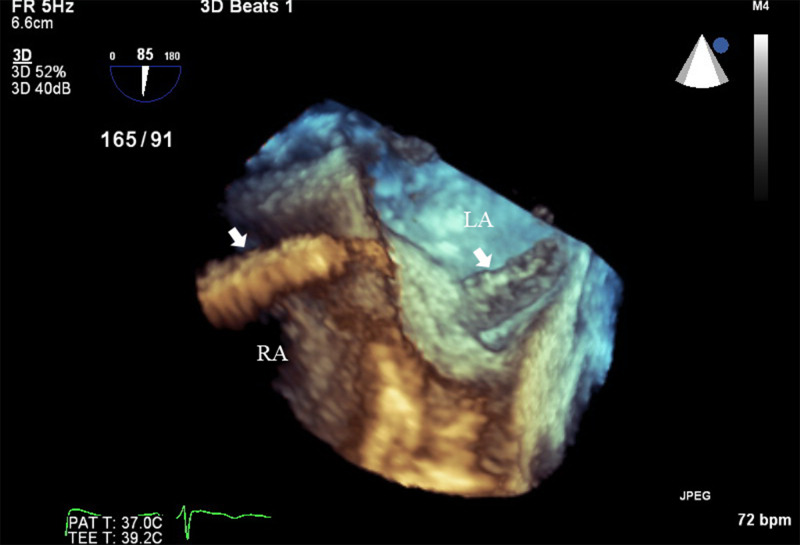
Transesophageal echocardiogram showing a three-dimensional image with thrombus (white arrow) migrating from right atrium to left atrium through patent foramen ovale. RA, right atrium; LA, left atrium.
